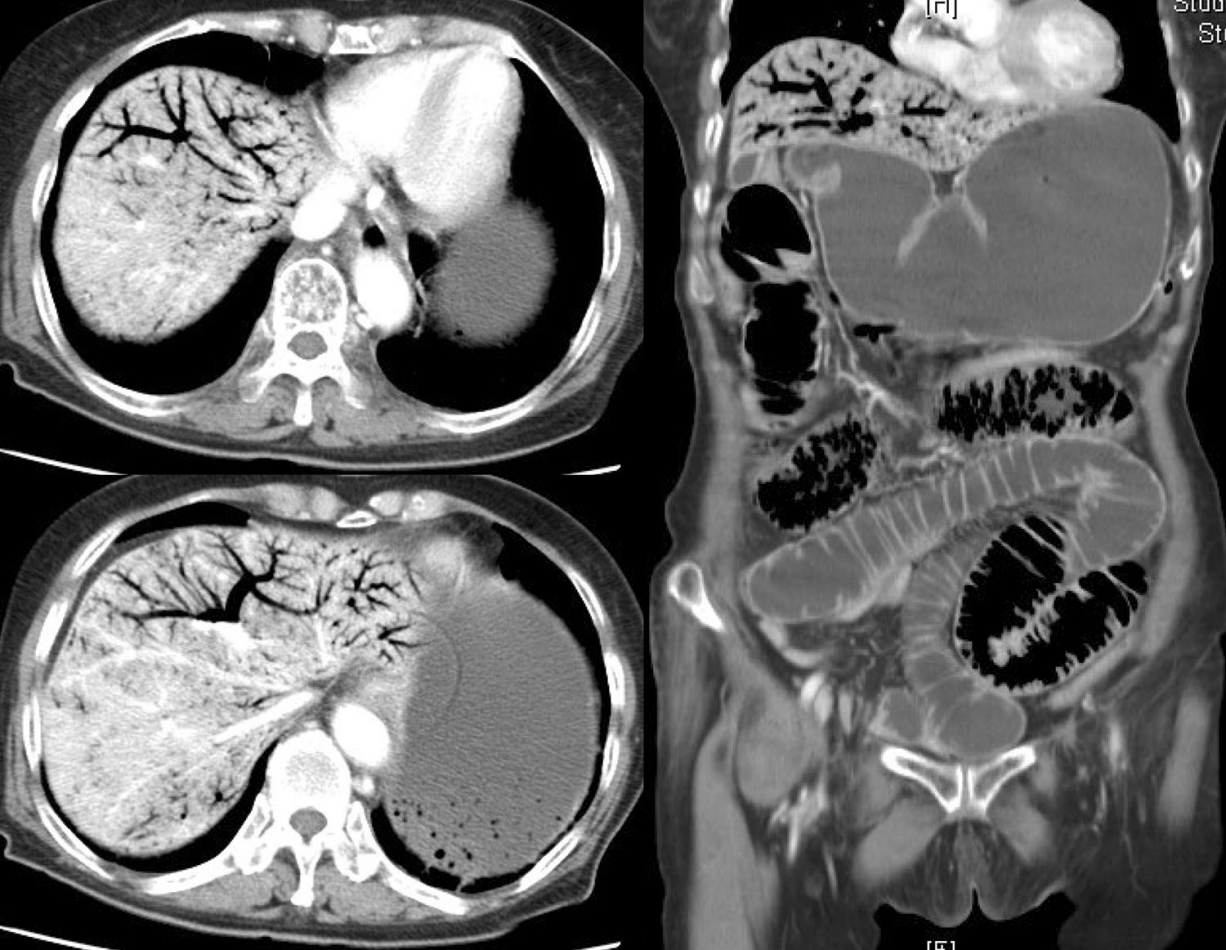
Multi-detector computed tomography (MDCT) findings of the patients with hepatic portal venous gas (HPVG)
